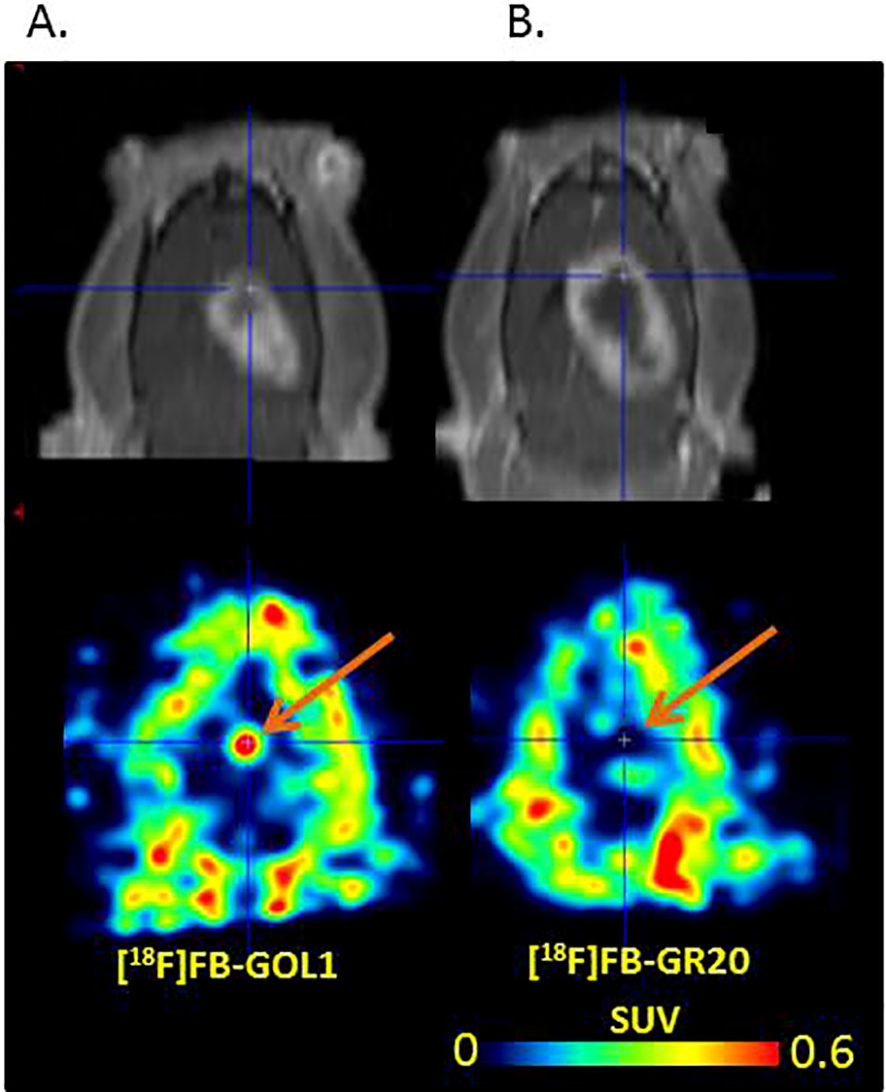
Formation of the intratumoral necrosis. Contrast enhanced T1-MRI combined with (a) [^18^F]FB-Gol1; (b) [^18^F]FB-GR20. The area of necrosis formation is pointed by the arrow on PET images.
